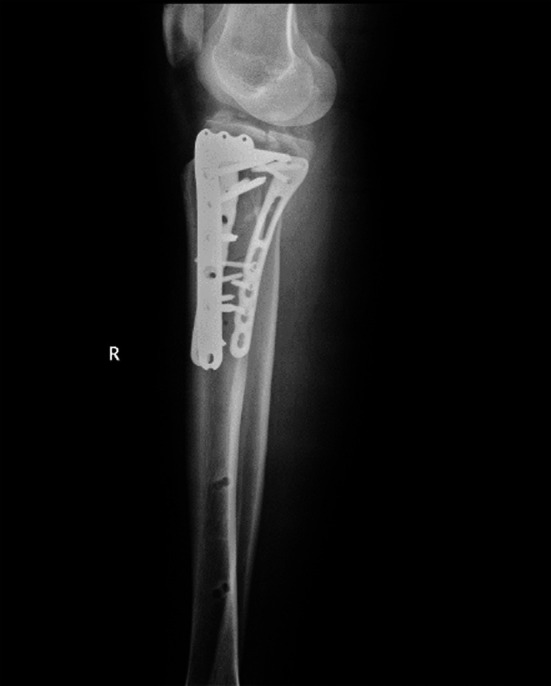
X-ray film of tibial plateau fracture after operation.
